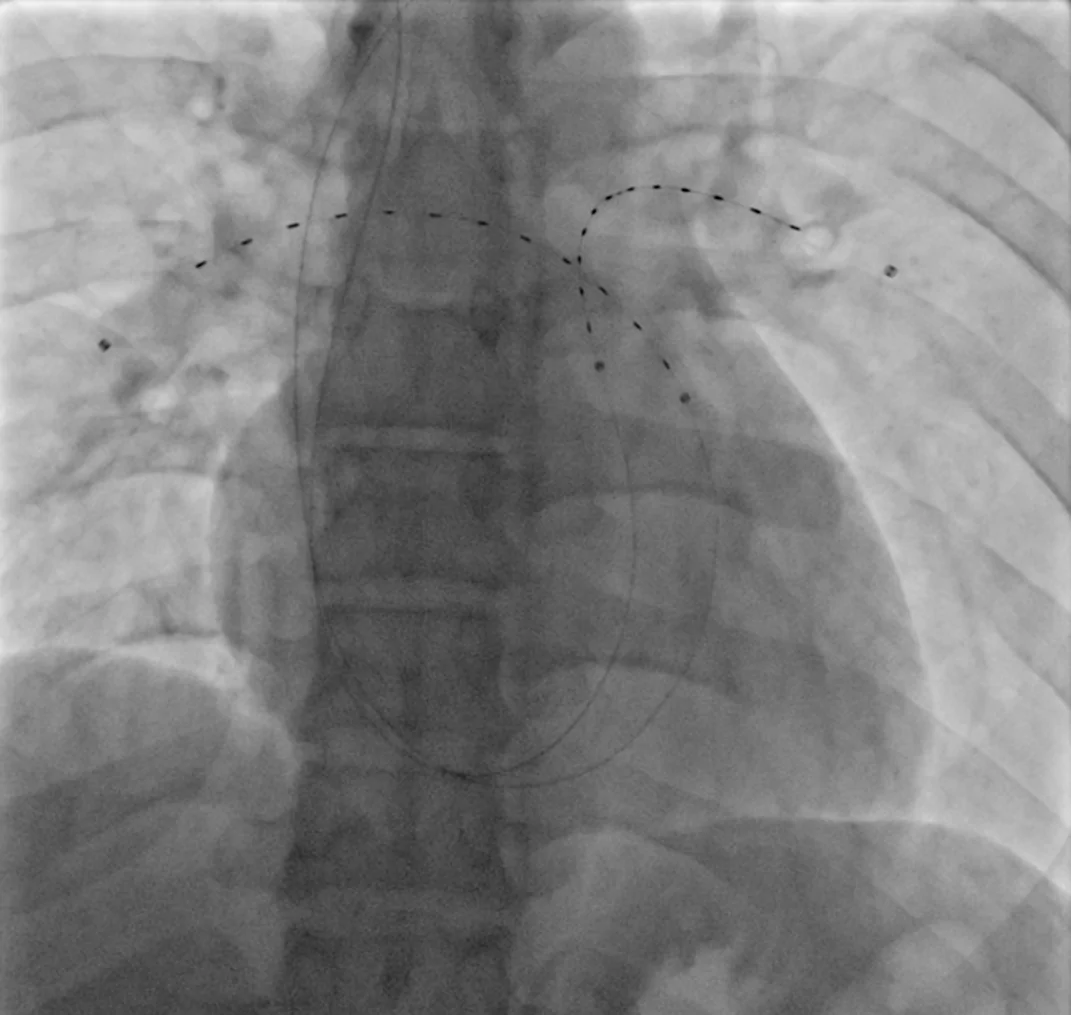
Fluoroscopic Images of Catheter-Directed Thrombolysis Using the EKOS Ultrasound-Assisted Catheter in Both Pulmonary Arteries
